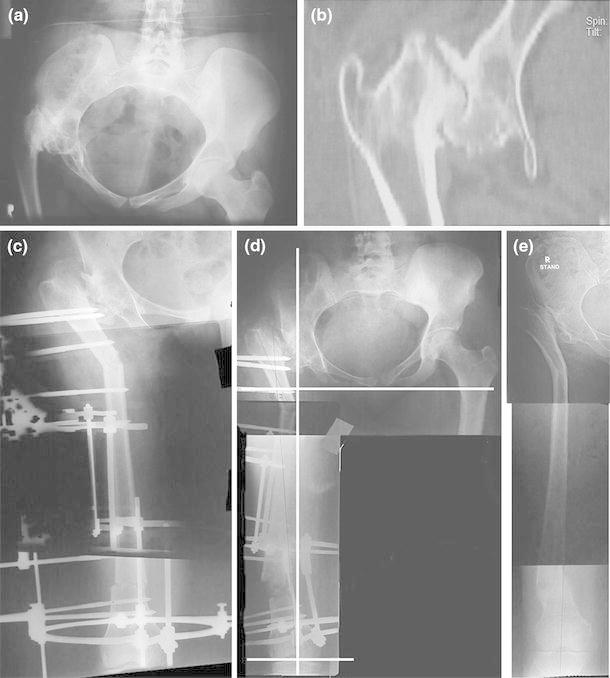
a, b Preoperative plain radiograph and CT scan show destruction of the femoral head and acetabulum and fibrous ankylosis as a result of septic arthritis of the right hip in childhood. The radiograph shows evidence of hip adduction, flexion (non-visualized obturator foramen) and internal rotation (non-visualized lesser trochanter). c, d Radiographs during treatment show the proximal and distal osteotomies, lengthening and varus through the distal osteotomy. The knee joint-orientation line is horizontal and proximal extension of the mechanical axis of the distal femur passes through the acetabulum. e Follow-up radiograph shows the consolidated osteotomies and maintained alignment
